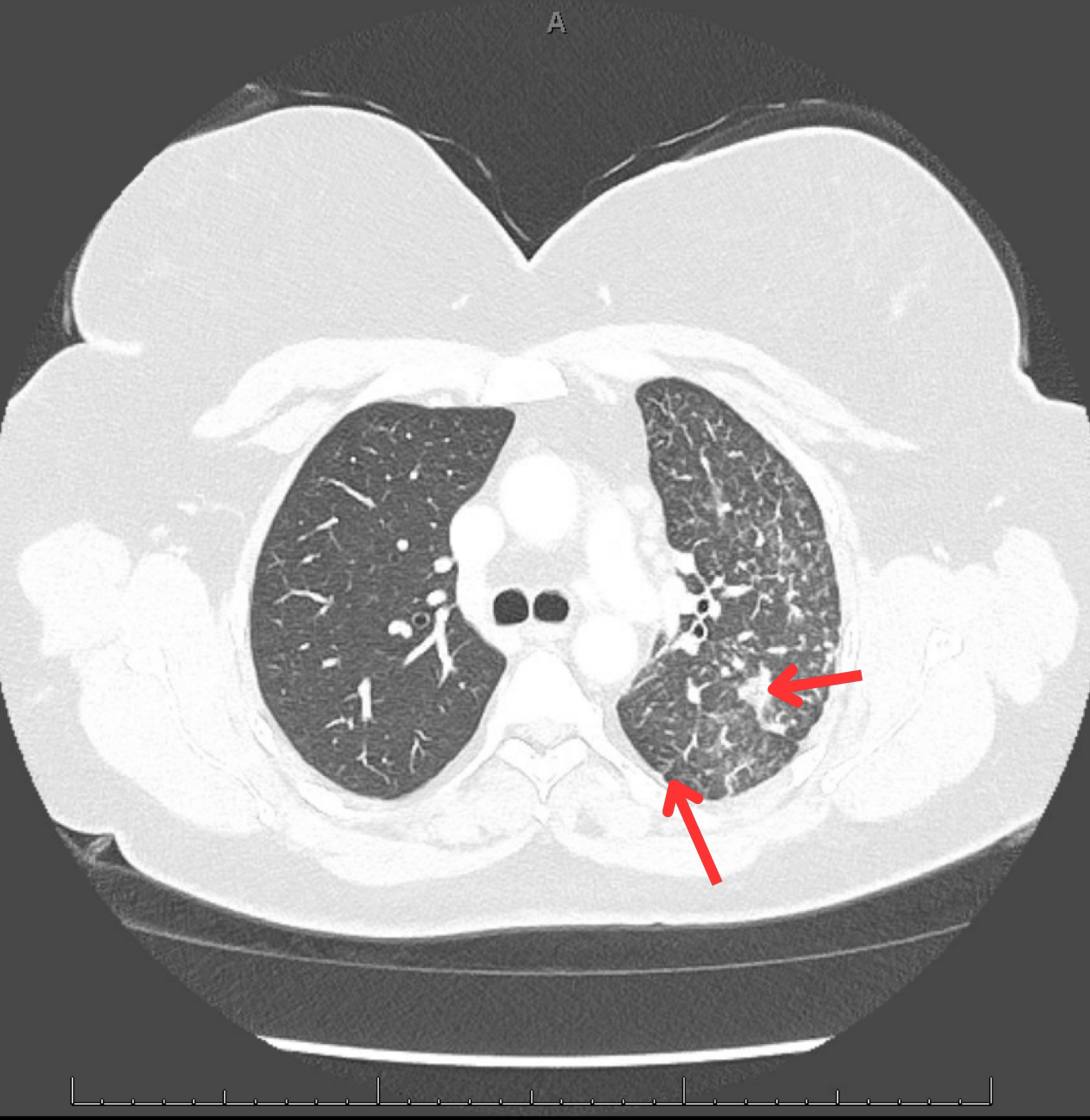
Axial CT image showing nodular infiltrates and interstitial thickening in the left lung showing patchy nodular infiltrates and interstitial thickening within the left lung. The opacities are predominantly peribronchiolar and subpleural in distribution, contributing to diagnostic ambiguity between infection and malignancy. The top red arrow indicates consolidative change, and the bottom red arrow shows linear opacity with distal airway plugging.
